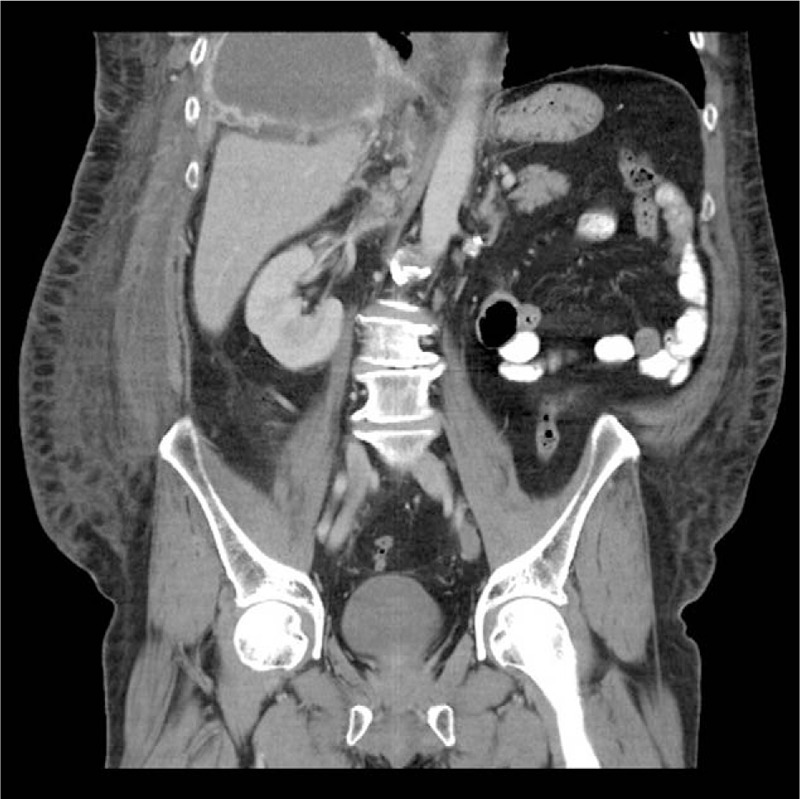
Sagittal contrast-enhanced computed tomography of lymphedema on the right side of the abdomen.
